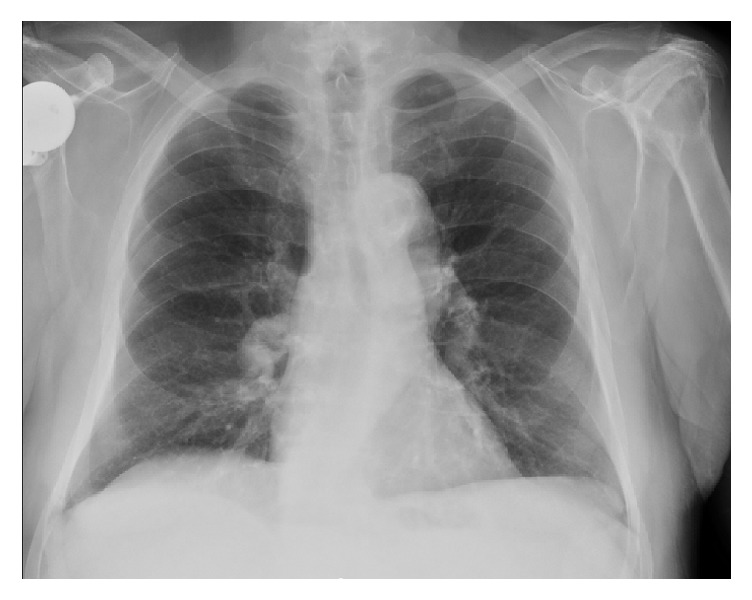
Chest X-ray 1 year postoperatively.
